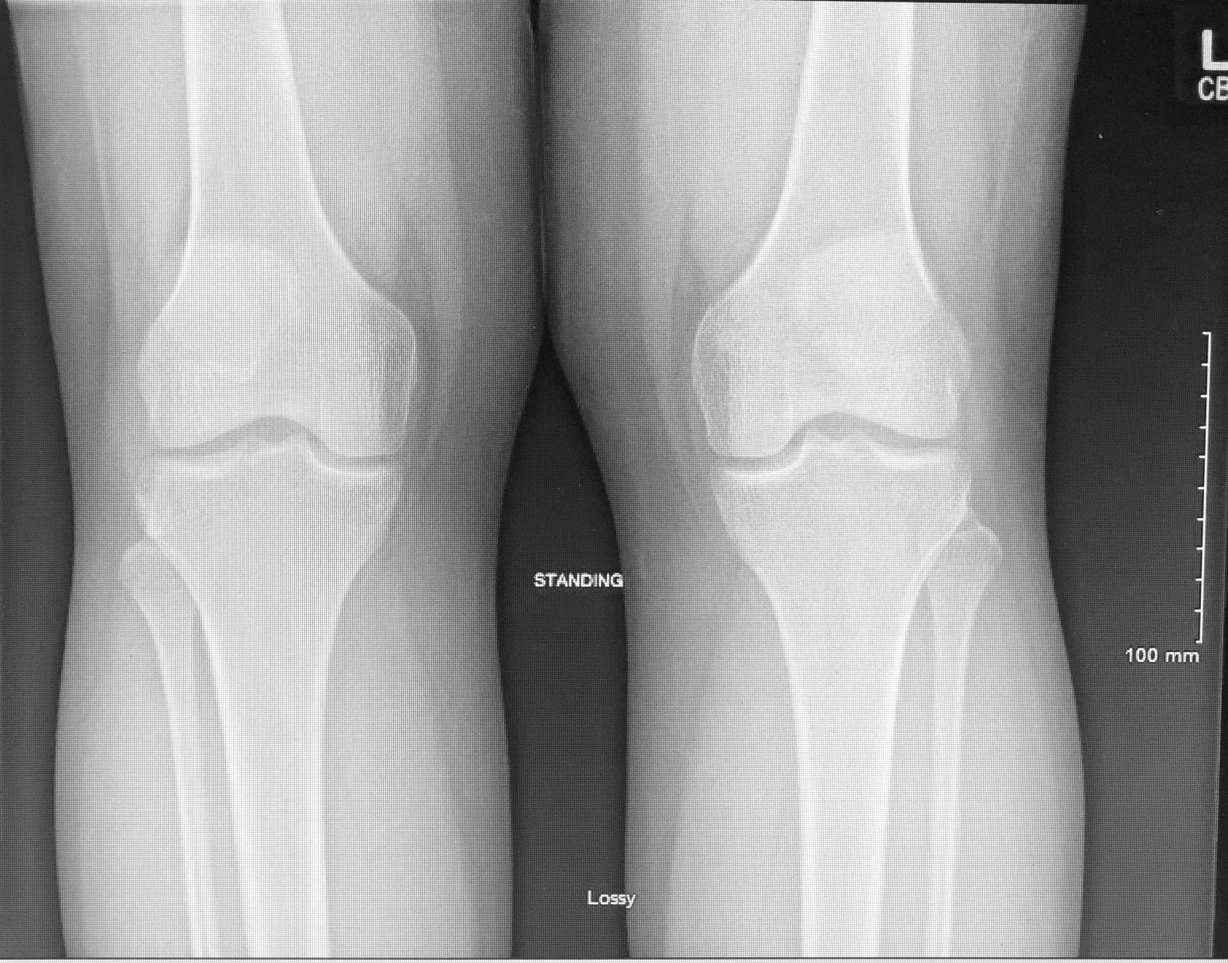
X-ray Bilateral Knee in Standing View
